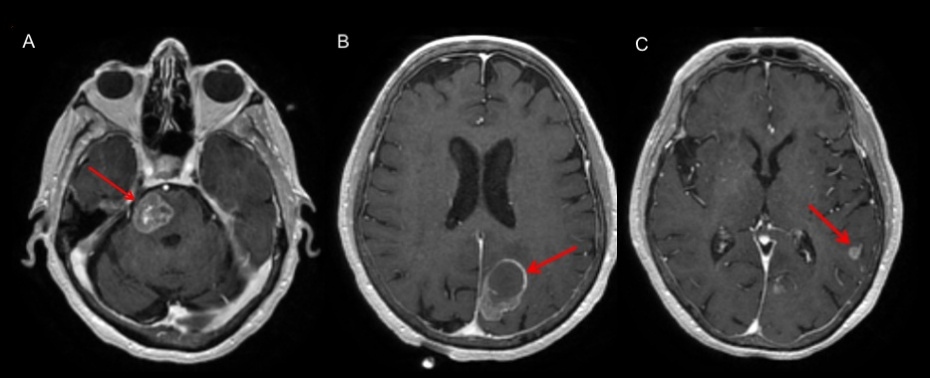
Representative MRI images at diagnosis, showing three brain lesions Axial images showing three heterogeneously enhancing lesions involving the (A) right pons, (B) the left inferior parietal lobe demonstrating a hemorrhagic component, and (C) the left supramarginal gyrus. MRI: magnetic resonance imaging
